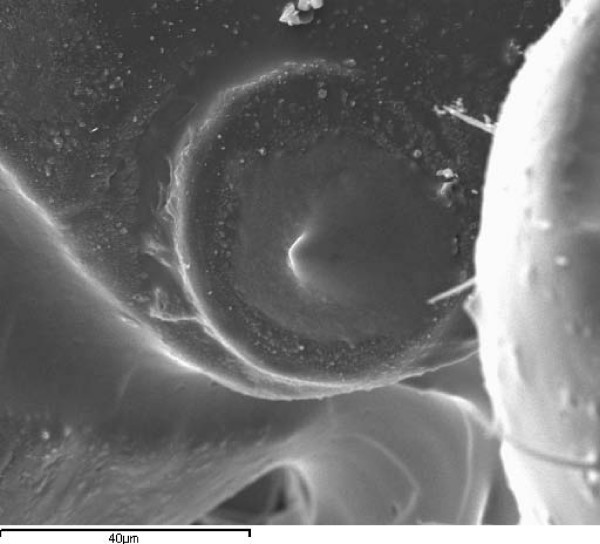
Treated abdominal spiracle occluded by a silicone plug. SEM of a treated spiracle in which the whole of the aperture is blocked by a silicone deposit. X-ray analysis showed that elemental silicon was present on the surrounding cuticle of the body, the peritreme of the spiracle, and the occluding plug across the spiracle opening.
